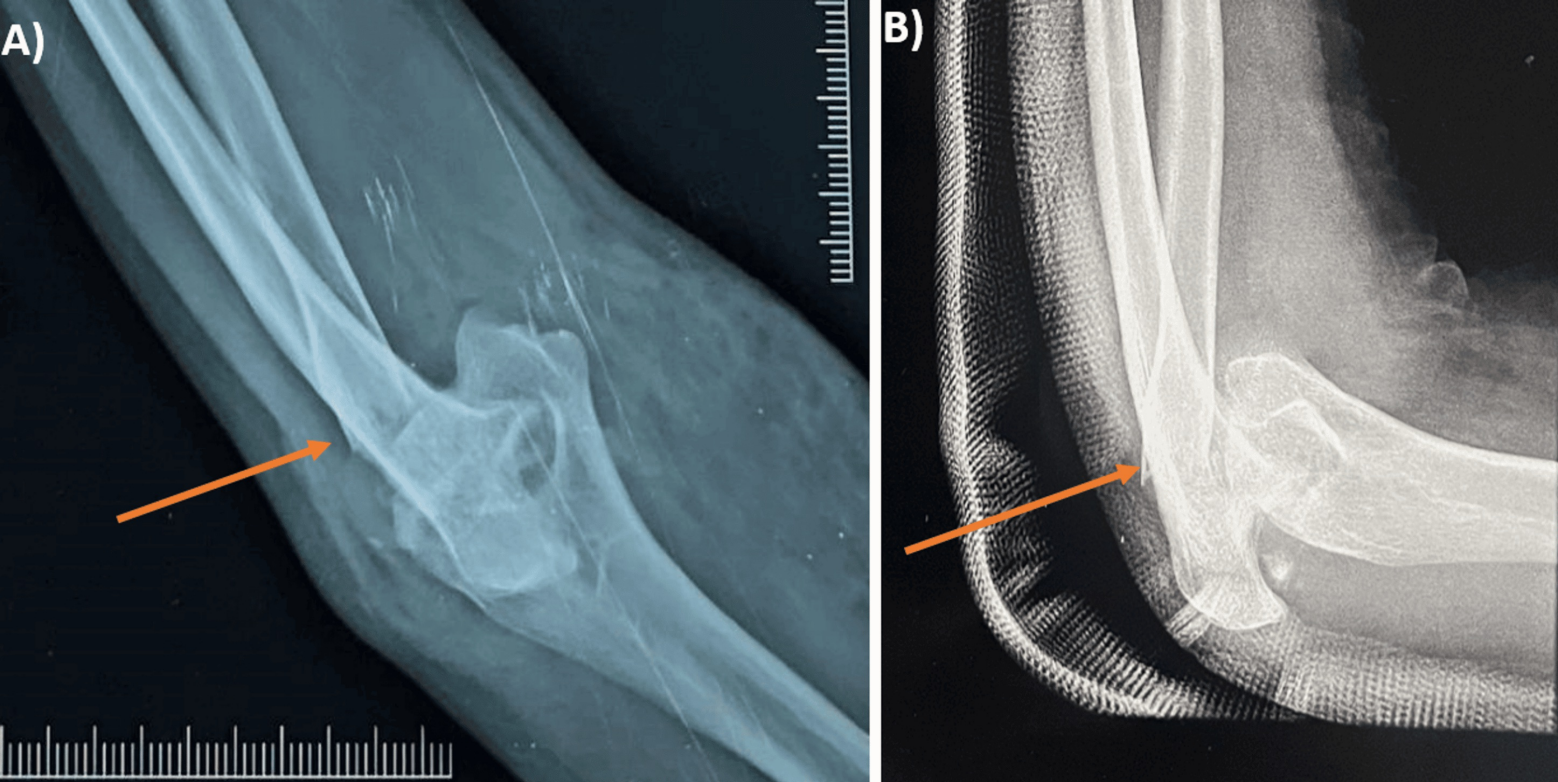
Preoperative X-ray A) Preoperative radiograph showing the anteroposterior (AP) view of the left elbow with Mason type IV radial head fracture. B) Preoperative radiograph showing the lateral view of the left elbow with Mason type IV radial head fracture.
